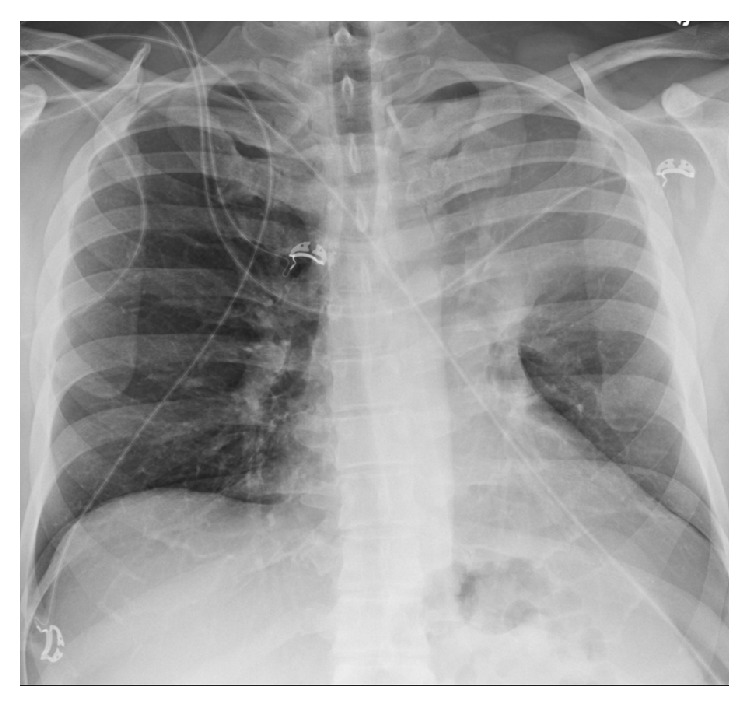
PA chest radiograph demonstrates complete left upper lobe atelectasis and the luftsichel sign.
